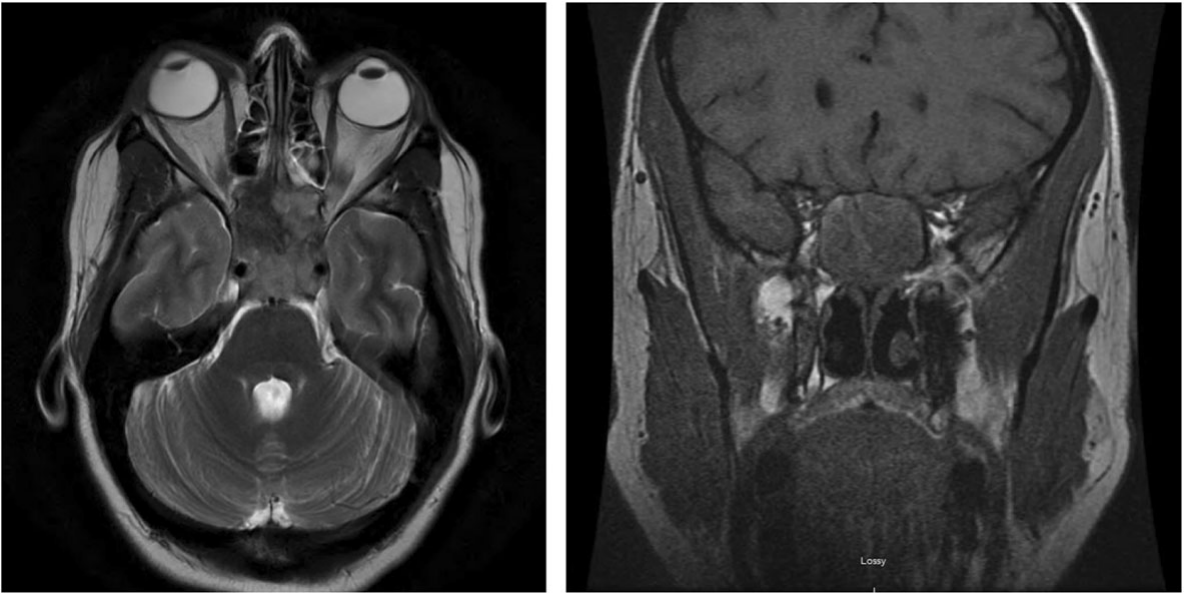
Magnetic resonance imaging demonstrating the identified 2.6 by 1.7 cm mass centered in the sella extending superiorly along the pituitary infundibulum, laterally into the cavernous sinuses, bilaterally to abut the carotid arteries, and anteriorly into the bilateral sphenoid sinuses with transverse (*left*) and coronal (*right*) planes shown
